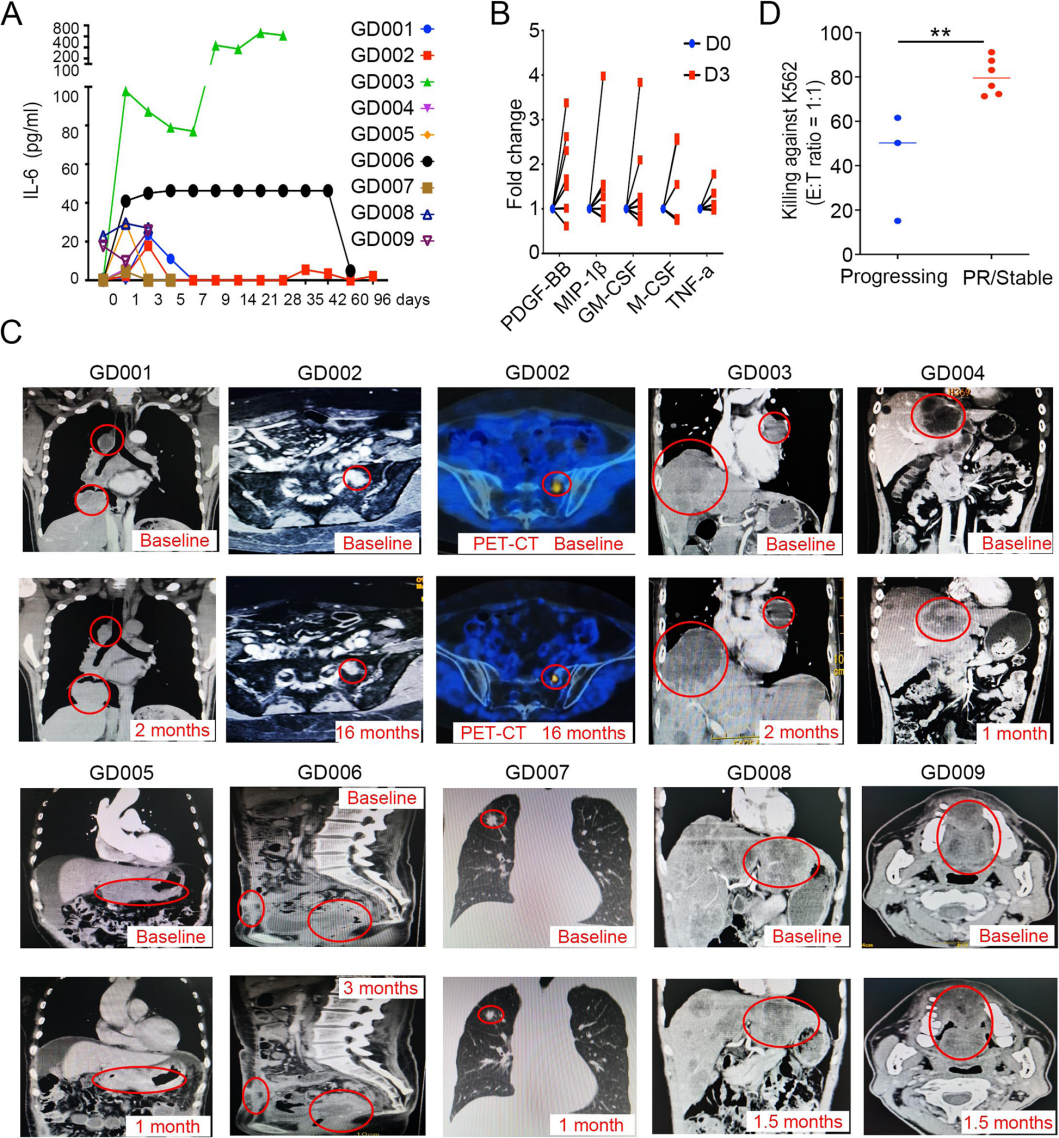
Safety and potency of ITNK infusions in cancer patients. A Serum IL-6 levels in patients (GD001-GD009) from day 1 to 3 months post ITNK infusion. B Cytokine levels in patients on day 0 and day 3 post ITNK infusion. PDGF-BB, MIP-1β, GM-CSF, M-CSF and TNF-α were significantly elevated in patients (GD002, GD004-GD007) after ITNK infusion on day 3 based on Benjamini–Hochberg-adjusted *p* value. C Representative CT scans and PET-CT (MRI) of patients before (baseline) and after ITNK infusion at indicated time points post initial ITNK infusion. Tumors in patients GD004, GD005, GD006, GD007 and GD008 were stabilized (all tumors increased less than 20% in size), and GD002 achieved partial remission (PR), as revealed by both CT and PET-CT scan imaging diagnostic analysis at 16 months (34.6% decrease in tumor size), whereas tumors in patients GD001, GD003 and GD009 progressed following ITNK treatment (all tumors increased more than 30% in size). Red circles indicate the monitored tumor sites. D Percentages of ITNK cytotoxicity for patients with progressing diseases and patients with stable diseases. The assay was performed against K562 cells at an E: T ratio equal to 1:1 measured 24 h after coculture. Data are shown as the mean ± SD; *P* = 0.0082 (stable vs. progressing); unpaired t test, *P* ≤ 0.01
